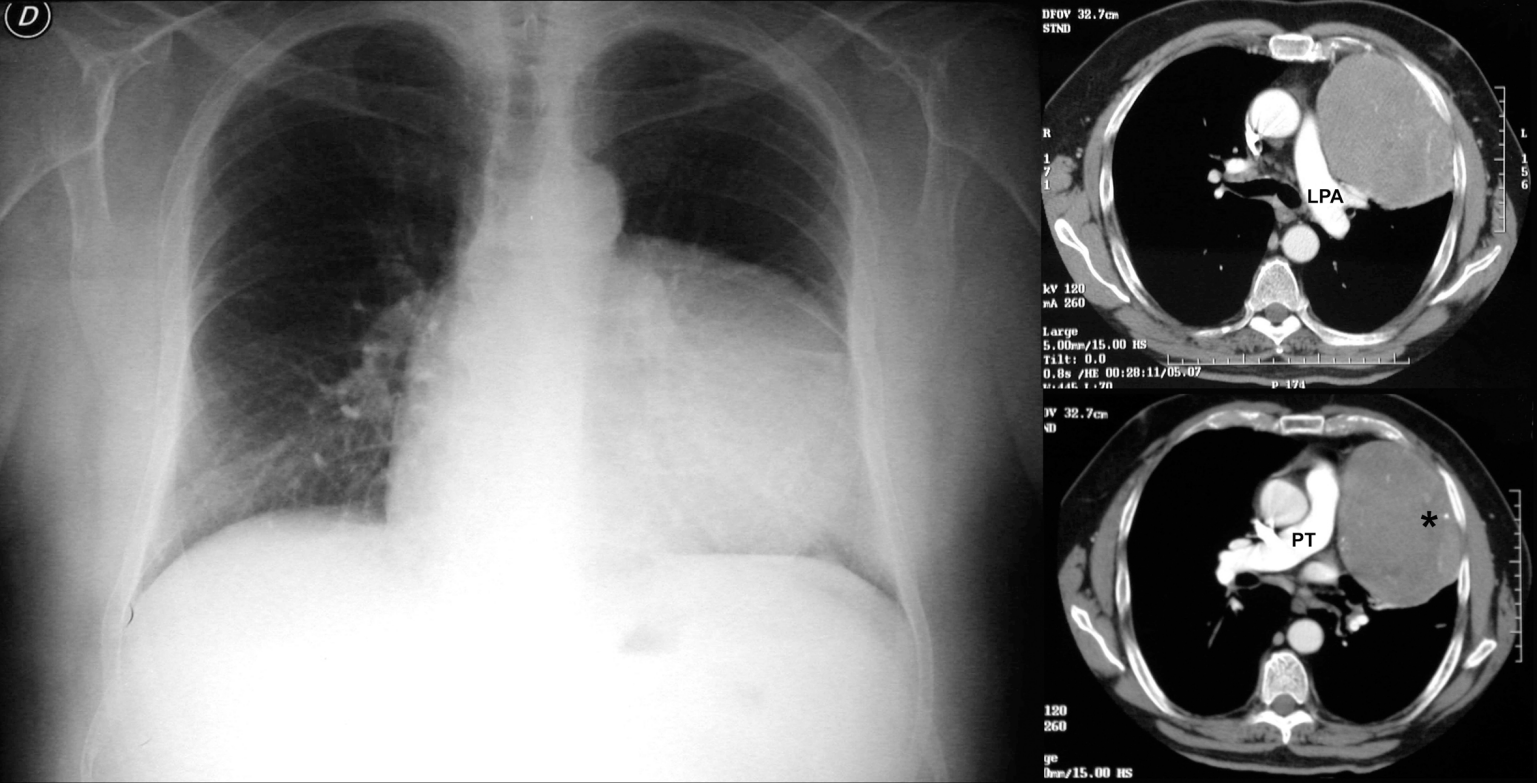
Left panel*: chest x-ray showing a large opacity on the left side. *Right panel*: the CT scan of the chest showing a solid mass of 11 × 8 cm in the left hemi thorax, with vertical extension of 10 cm, mild enhancement after contrast medium infusion and some calcifications in the basal part (asterisk). The lesion is in close relation with chest wall, left pulmonary artery (LPA) and pulmonary trunk (PT), without signs of local infiltration.
